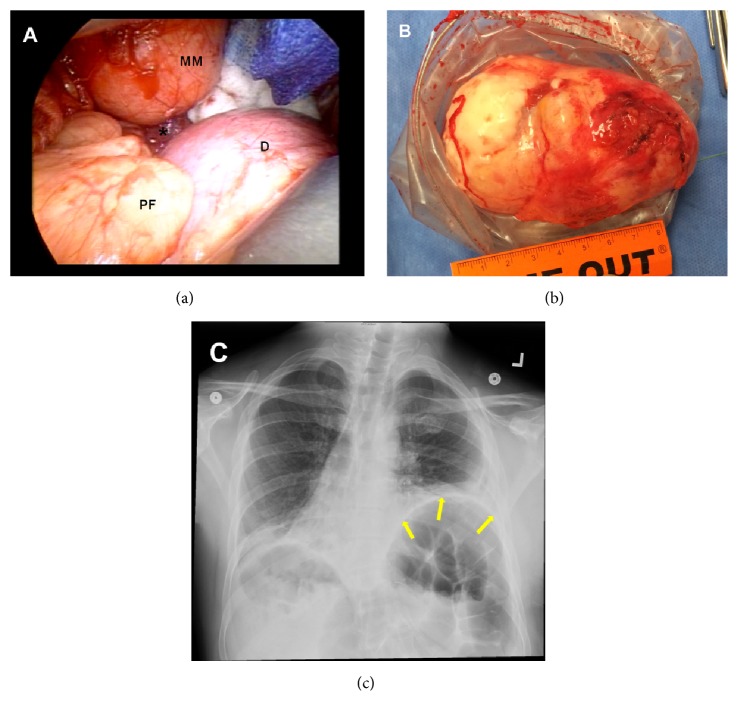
(a) Left thoracotomy/thoracoscopic view of the mass (MM) atop of the diaphragm (D) anterior to the left lung (asterisk) (PF, pericardial fat pad). (b) Gross view of the intact excised mass measuring 10 × 6 × 4.5 cm. (c) Postoperative chest X-ray showing resolution of the left sided mediastinal mass, with no change in the hemidiaphragm (yellow arrows) and the right sided heart.
